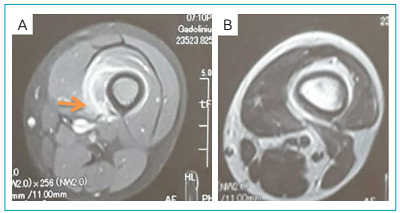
Magnetic resonance imaging showing signs of osteomyelitis. (A and B): MRI with contrast shows signal enhancement of the left femur with soft tissue signal abnormality, suggestive of osteomyelitis.
